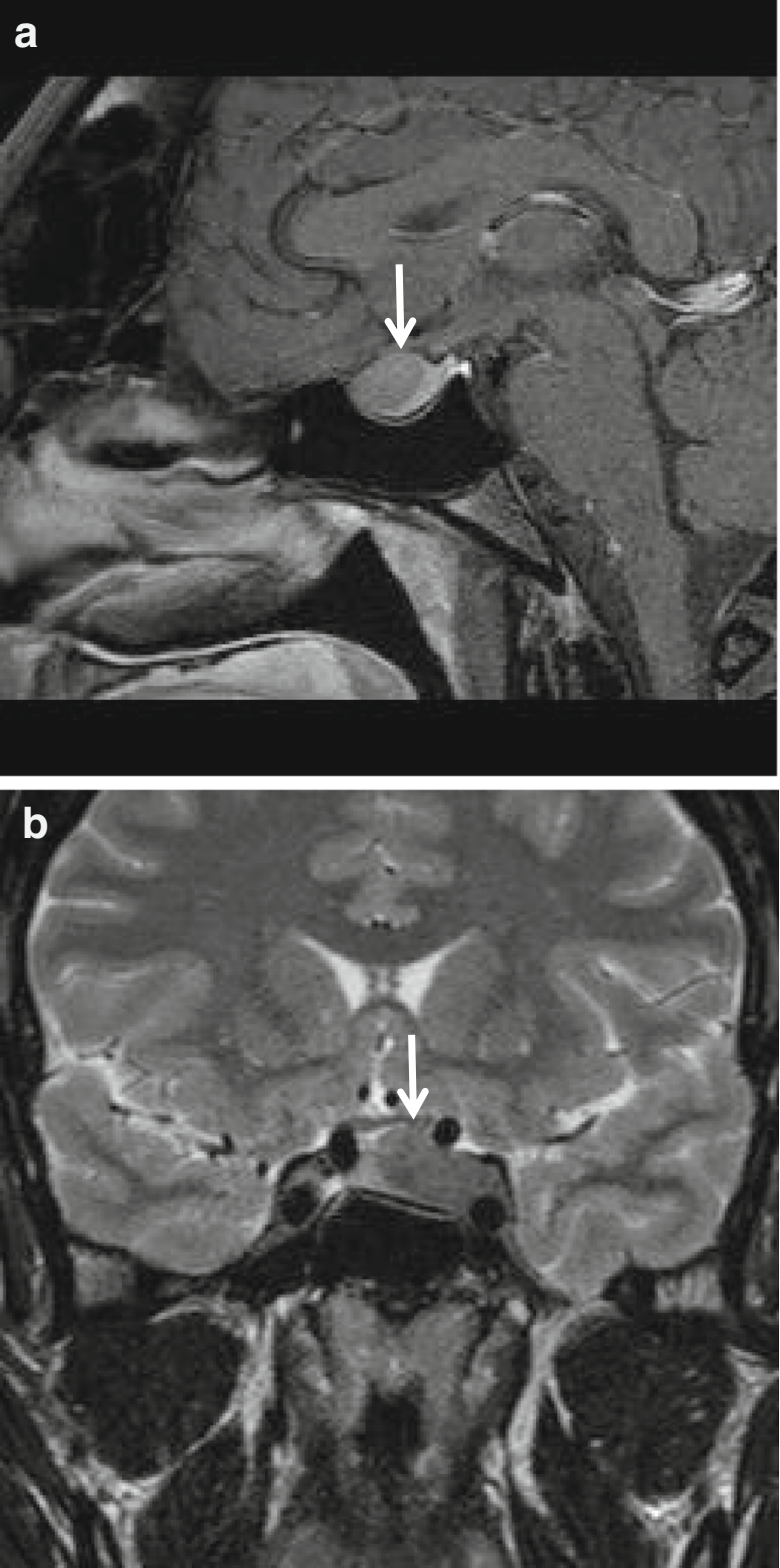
Magnetic resonance imaging of brain. a Sagittal section, and b coronal section; *arrow* indicates pituitary tumor
